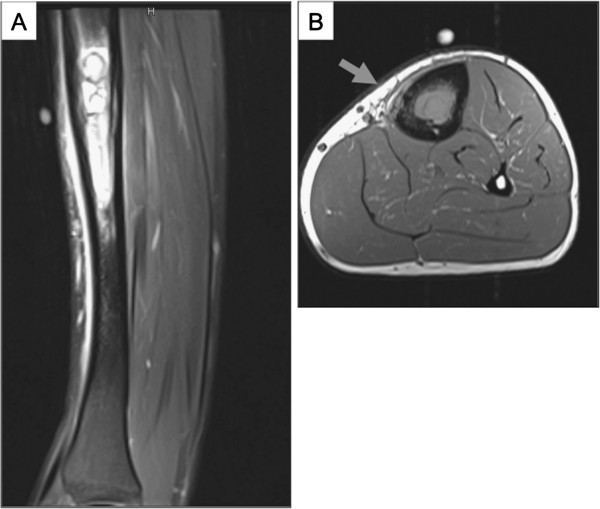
Case 2: (A) Sagittal image showing a high-signal medullary lesion of the tibial diaphysis. The linear high signal anterior to the tibia represents reactive oedema. (B) T1-weighted axial image showing intramedullary tumour with focal cortical erosion and a minor soft tissue component (arrowed).
